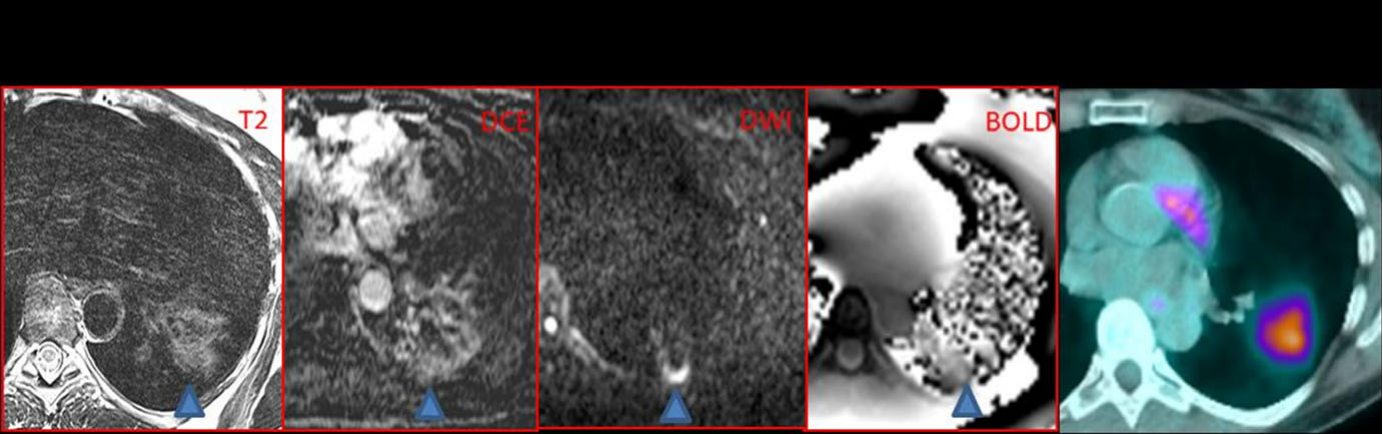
Different sequences provide different information. These parameters can be combined with the results found from positron emission tomography/magnetic resonance imaging (PET/MRI) (right image), or PET/MRI (not shown) to give an “in vivo” biology of tumor heterogeneity. [Color figure can be viewed at http://wileyonlinelibrary.com]
